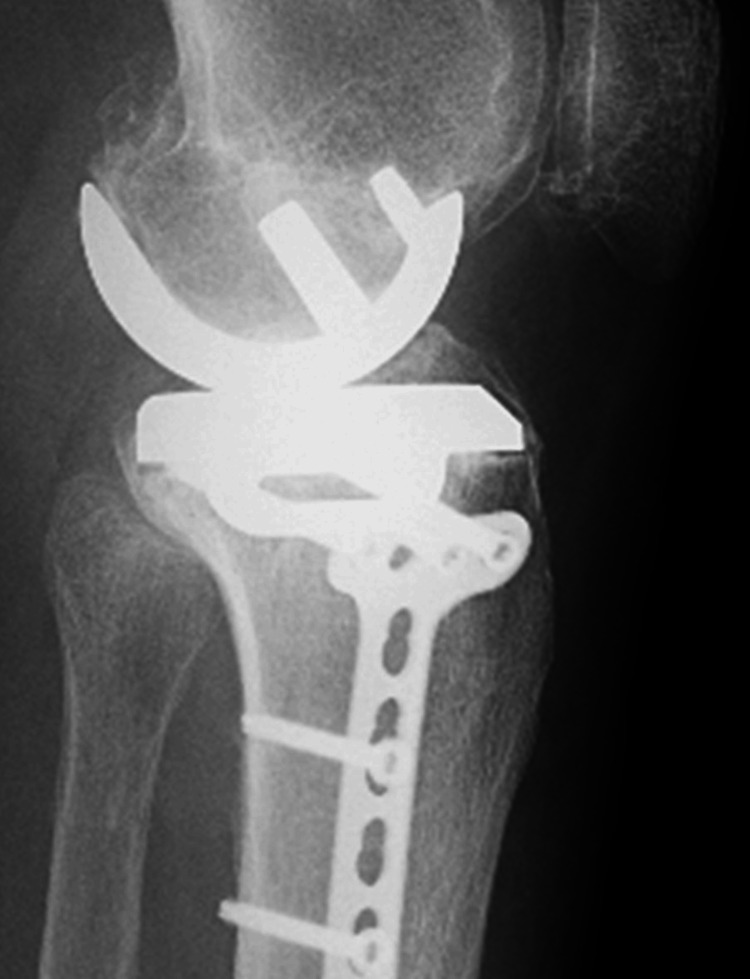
Radiography of reoperation with tibial compression plate (lateral view)
